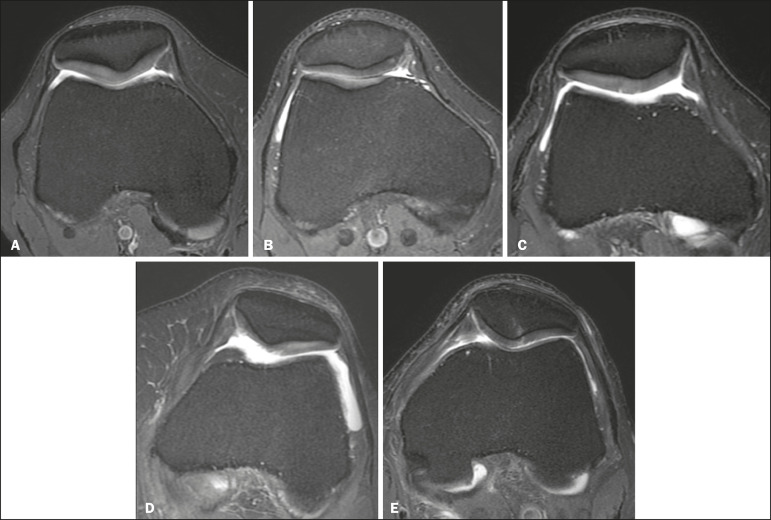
MR images according to the ICRS grades: normal examination (A), grade 1 (B), grade 2 (C), grade 3 (D), and grade 4 (E).
